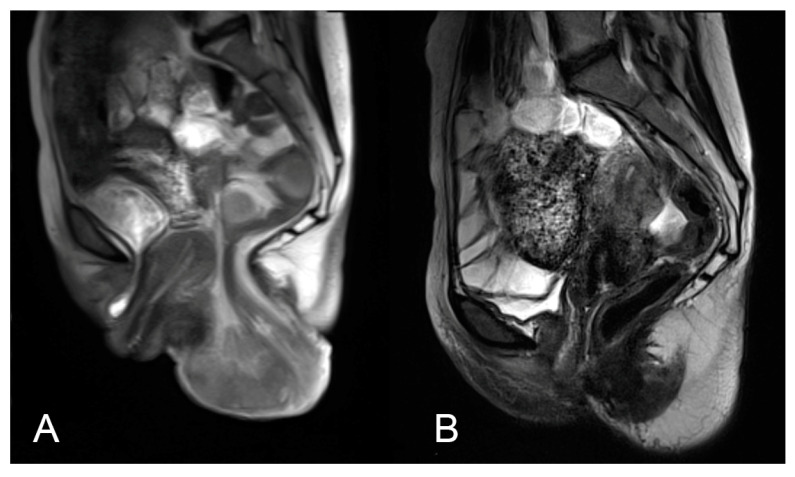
MRI of pelvic organs in sagittal view. (A) Total uterine and rectal prolapse at the time of admission in November 2017. (B) Final outcome after treatment in April 2018. This anatomical picture remains stable as of December 2024.
